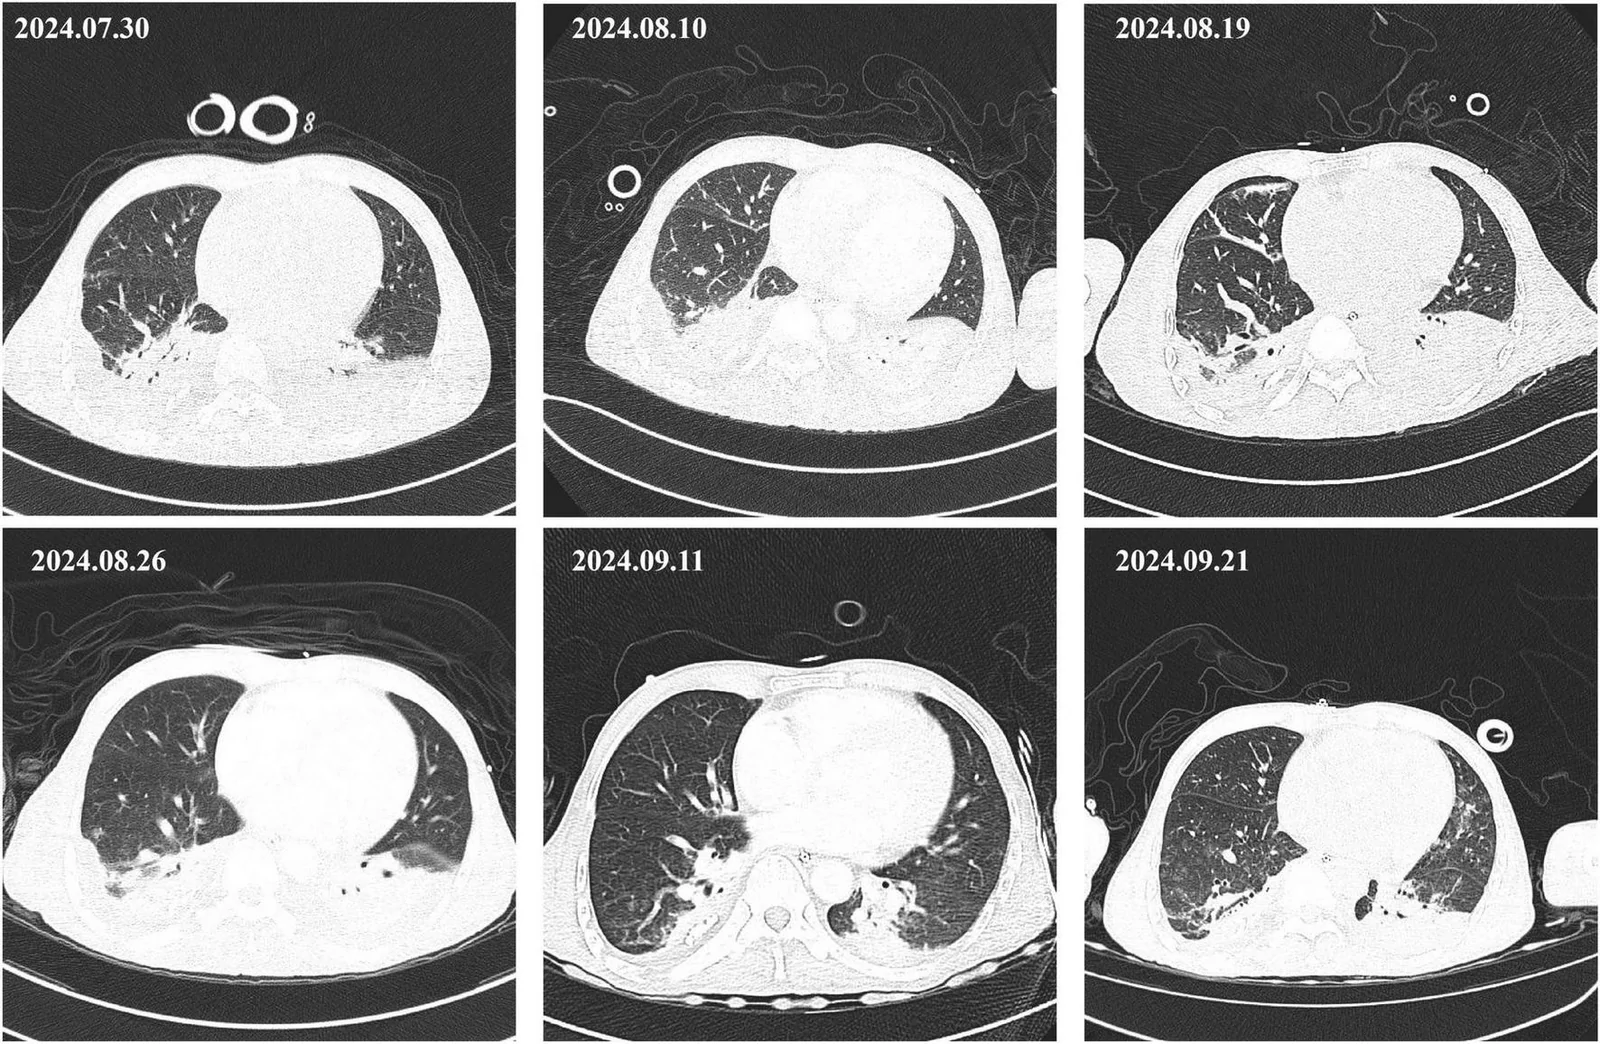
Serial chest CT images demonstrate gradual resolution of bilateral pulmonary infiltrates and pleural effusion over the 65-day hospitalization.
